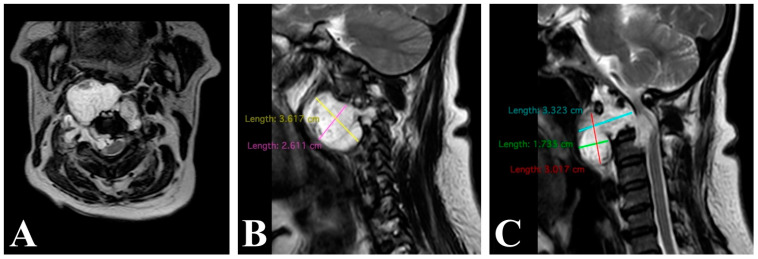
Axial (A) and sagittal (B,C) gadolinium-enhanced T1-weighted cervical spine MRI show C1–C2 lesion with more than 80% of spinal cord compression.
